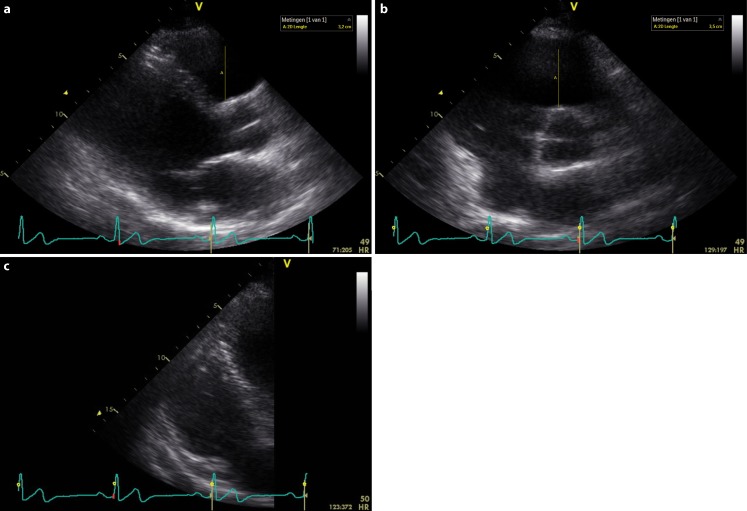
Echocardiogram in 2008 showing dilatation of the right ventricle and right ventricular outflow tract. a PLAX RVOT, b PSAX RVOT, c AP4CH-focused RV. *PLAX* parasternal long axis view*, RVOT* right ventricular outflow tract,* PSAX* parasternal short axis view*, AP4CH* apical 4 chamber view,* RV* right ventricle
